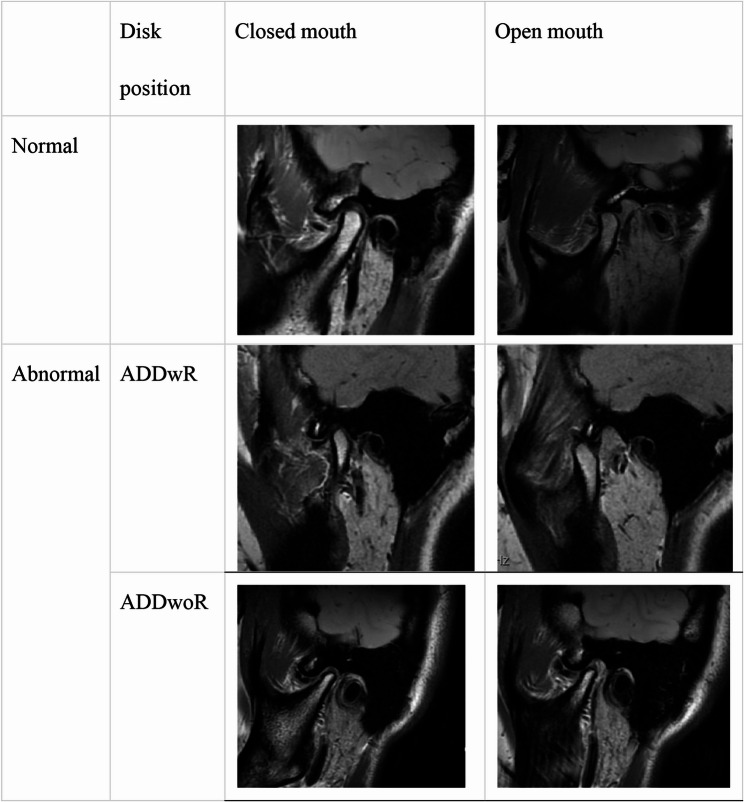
Magnetic resonance imaging (MRI) of normal and abnormal anterior disc locations with and without reduction. The posterior band of the joint disc is located at the top of the condyle. If the posterior band of the joint disc is located at 12 o’clock between the condyles, the joint disc position is considered normal. A disc located below 11 o’clock is considered anteriorly displaced. In the open oblique sagittal position, if the disco-condylar relationship returns to normal, it is reversible anterior disc displacement. If the condylar position of the articular disc cannot be restored in the open oblique sagittal position, it is irreversible anterior disc displacement
